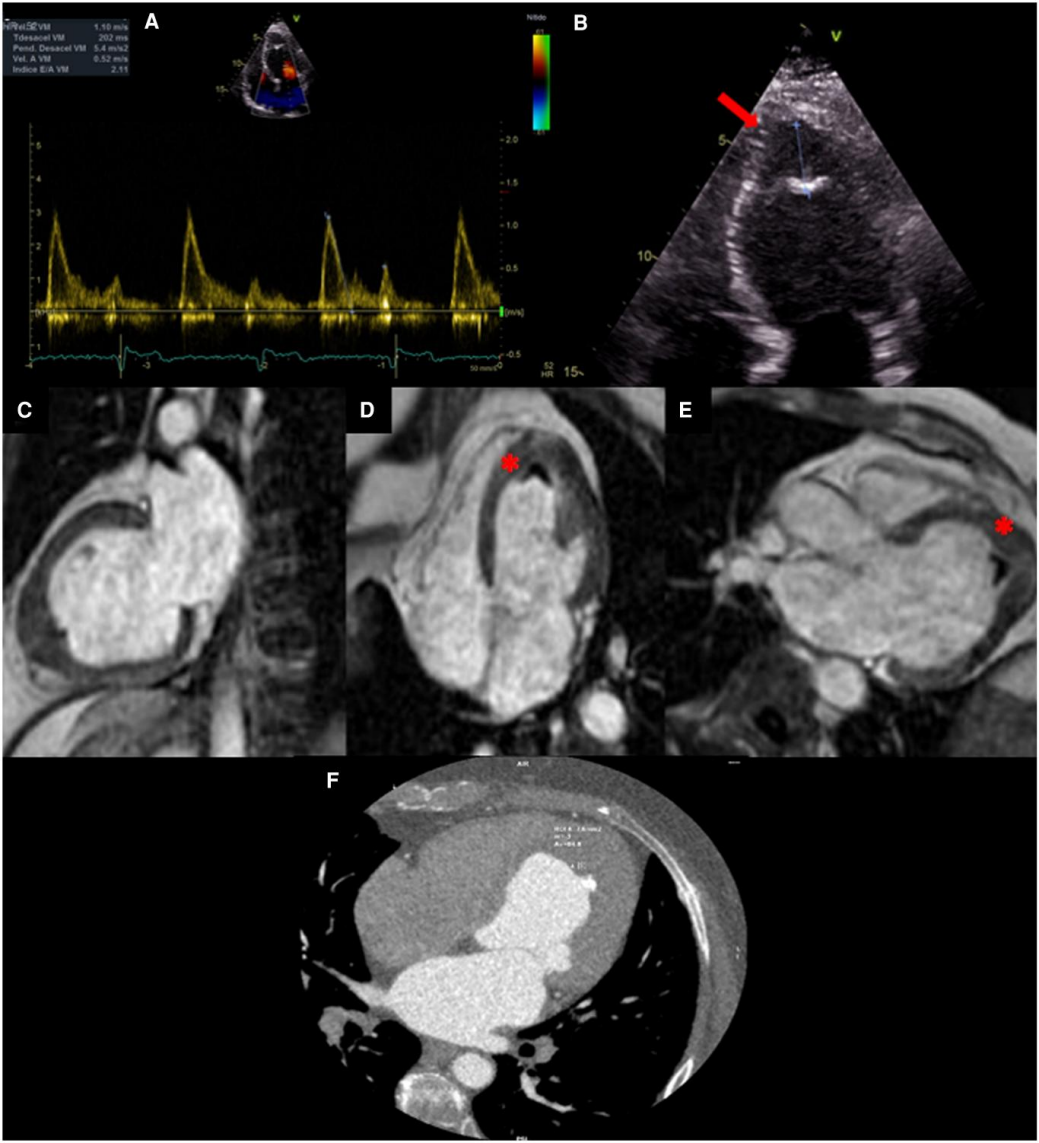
Case 1 images. (*A*) Pulsed Doppler demonstrates a restrictive filling pattern. Tissue Doppler showed a septal e′ wave at 5 cm/s. (*B*) Echocardiogram shows apical thickening with wedge-shaped internal calcification (arrow). In the lower row, we observe cardiac magnetic resonance in late gadolinium enhancement sequence. Two-chamber view (*C*) showing apical diastolic thickening and four- (*D*) and three-chamber views (*E*) showing apical endocardial enhancement with superimposed thrombus ‘double V sign’ (asterisk). (*F*) Cardiac computed tomography reformat four chambers showing apical obliteration with hypoattenuated image (85UH) and calcification.
